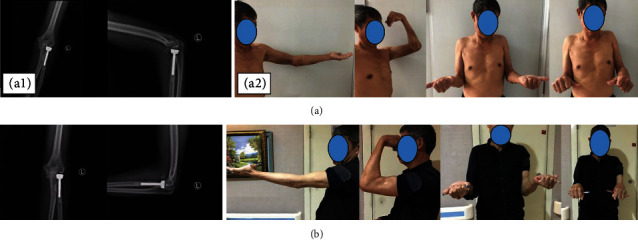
(a-1) X-ray films one month after removing the device show that the elbow joint alignment is normal, and there are no abnormalities such as dislocation. (a-2) Elbow extension function, elbow flexion function, forearm supination function, forearm pronation function, and good elbow stability one month after removal. (b-1) X-ray films four months after removal show that the elbow joint alignment is normal, and the radial head prosthesis is not loose or falling off. (b-2) Elbow extension function, elbow flexion function, forearm supination function, forearm pronation function, and good elbow stability four months after removal.
